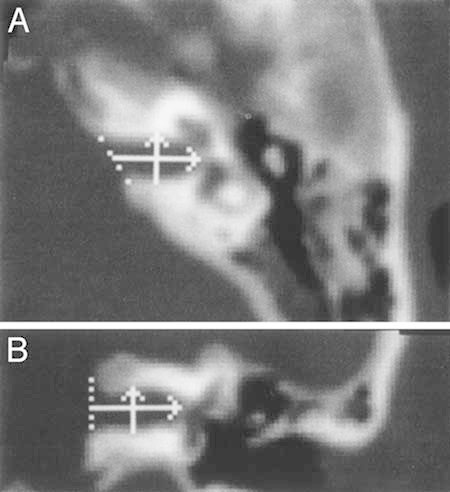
(A ) Axial and (B ) coronal computed tomography images of typical pagetic temporal bone showing placement of lines for dimensional measurement. 15 (Monsell EM, Bone HG, Cody DD, Jacobson GP, Newman CW, Patel SC, Divine GW. Hearing loss in Paget's disease of bone: evidence of auditory nerve integrity. *Am J Otol* 1995;16:27–33.)
